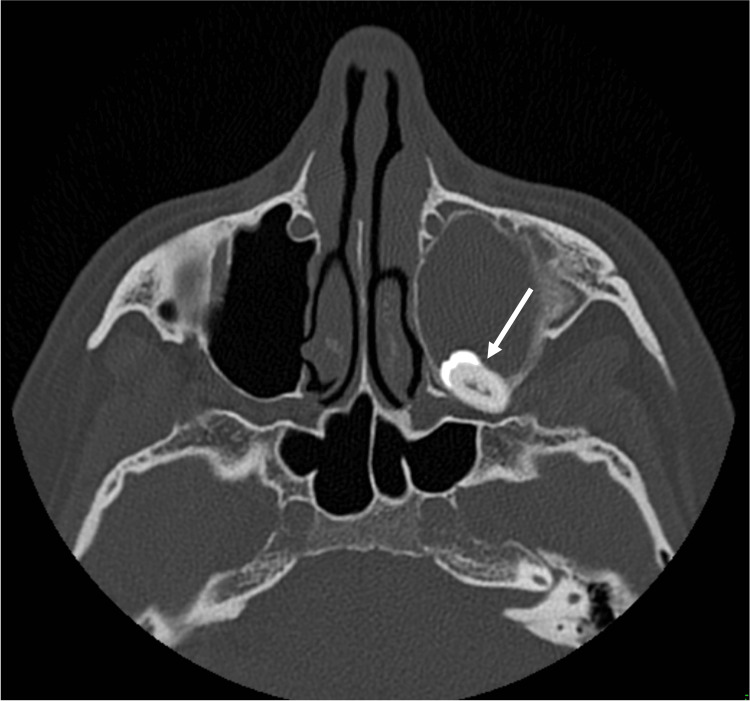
Preoperative maxillofacial CT scan Axial view of preoperative maxillofacial CT scan showing an isodense area with complete obliteration of left maxillary sinus and an ectopic tooth (arrow) in the posterior portion of the roof of the sinus
